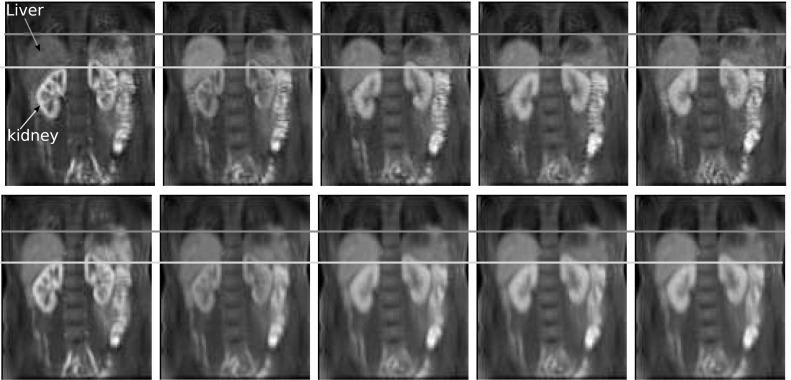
(*Top*) 5 frames at time = {30,50,74,82,100}s selected from a DCE-MRI sequence of a healthy volunteer. The *yellow* and *red reference lines* show the alignment of the kidney and liver regions. The *first image* shows the peak stage of contrast agent inside the kidney region. The regions of kidney and liver clearly depict the translation of movements in the vertical direction, arising due to the patient movements. (*Bottom*) The *yellow* and *red reference lines* clearly showing the proper alignment of the kidney and liver regions after processing with WR-DMD. The video-based results can be viewed at https://youtu.be/TWq34TFGNcU and https://youtu.be/UT7f4ch4H-I (colour figure online)
